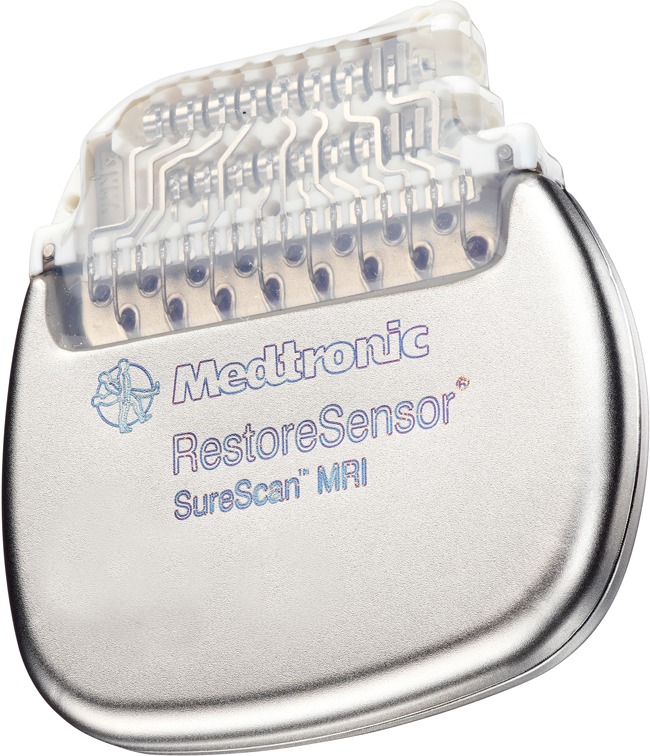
The Medtronic RestoreSensor SureScan MRI SCS device (Medtronic, Minneapolis, MN, USA). Reprinted with the permission of Medtronic, Inc. © 2013
